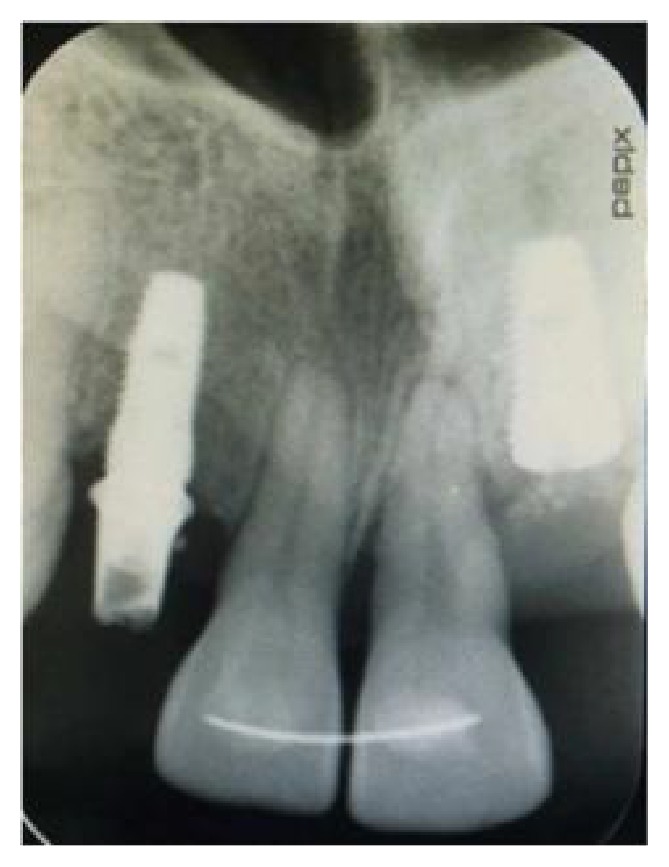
A radiograph showing the 2 implants replacing the teeth 12, 22.
